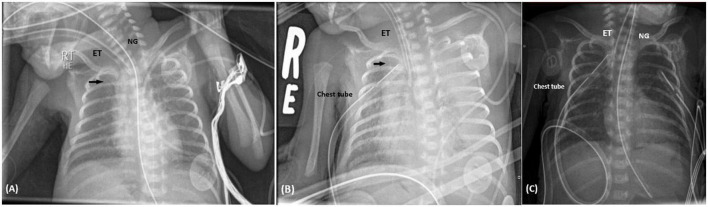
(A) Supine chest radiograph following PICC insertion in Case I. Chest X-ray performed in supine position, revealed the PICC inserted through the right basilic vein reaching the left subclavian vein. According to this image, the PICC was retracted 2.5 cm. The black arrow identifies the PICC. NG, nasogastric tube; ET, endotracheal tube. (B) Supine chest radiograph following the sudden deterioration in clinical status. Chest X-ray was carried out when the patient had sudden cardiopulmonary decompression. It revealed total whitening of the left lung, as shown on the left side of the image, representing total collapse with left sided pleural effusion. The PICC is represented by the black arrow. ET, endotracheal tube. (C) Chest imaging 2 days following chest tube insertion. Chest X-ray showed complete resolution of the pleural effusion, and normal aeration of the left lung. NG, nasogastric tube; ET, endotracheal tube.
